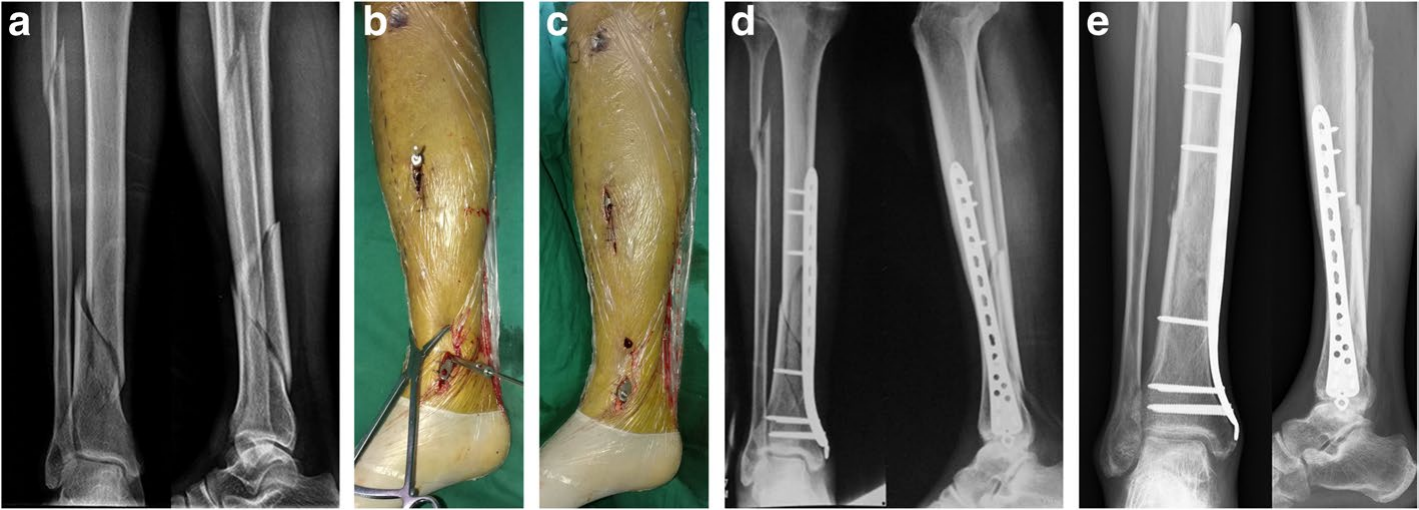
A–E Reduction of a distal tibia fracture using manual traction. A Anteroposterior and lateral radiographs of a male who sustained an AO 43-A2.3 type distal tibia fracture. B A manual traction reduction technique was used to achieve indirect reduction. C Final view of operated limb after completion of MIPO. D Postoperative anteroposterior and lateral radiographs. E Anteroposterior and lateral radiographs taken 8 months postoperative demonstrate a healed distal tibia
